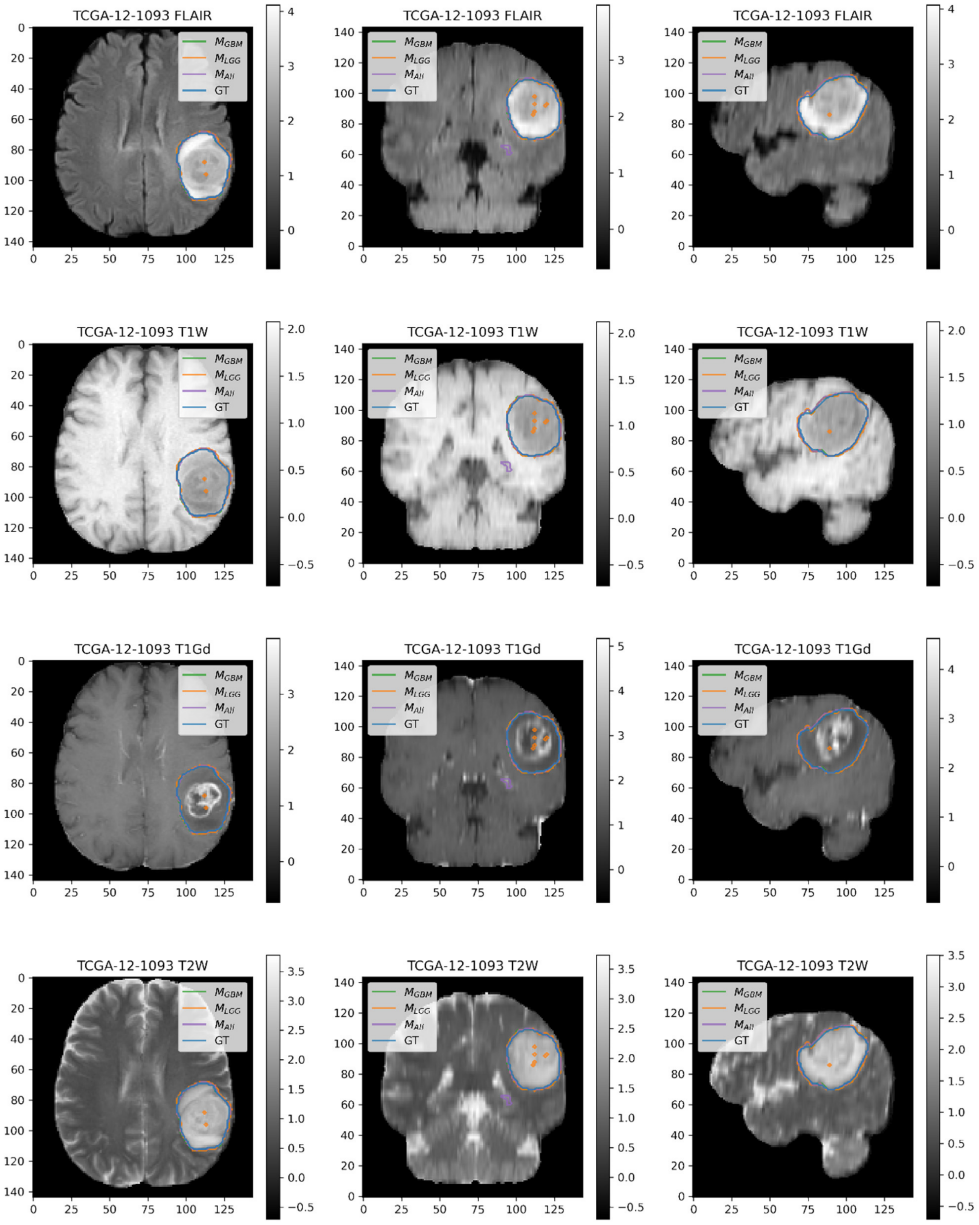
Patient TCGA-12-1093 belongs to *D*_*GBM*_ and has a tumor in the left parietal lobe. We selected this patient as an example because it has consistently good performance for metrics (Sens, B.Acc, Dice, Jac.C) across all models. This GBM has clear margins, and a sharp boundary on FLAIR enhancing regions. The enhancing tumor core is central and distinct, and the models all perform relatively consistently in segmentation.
